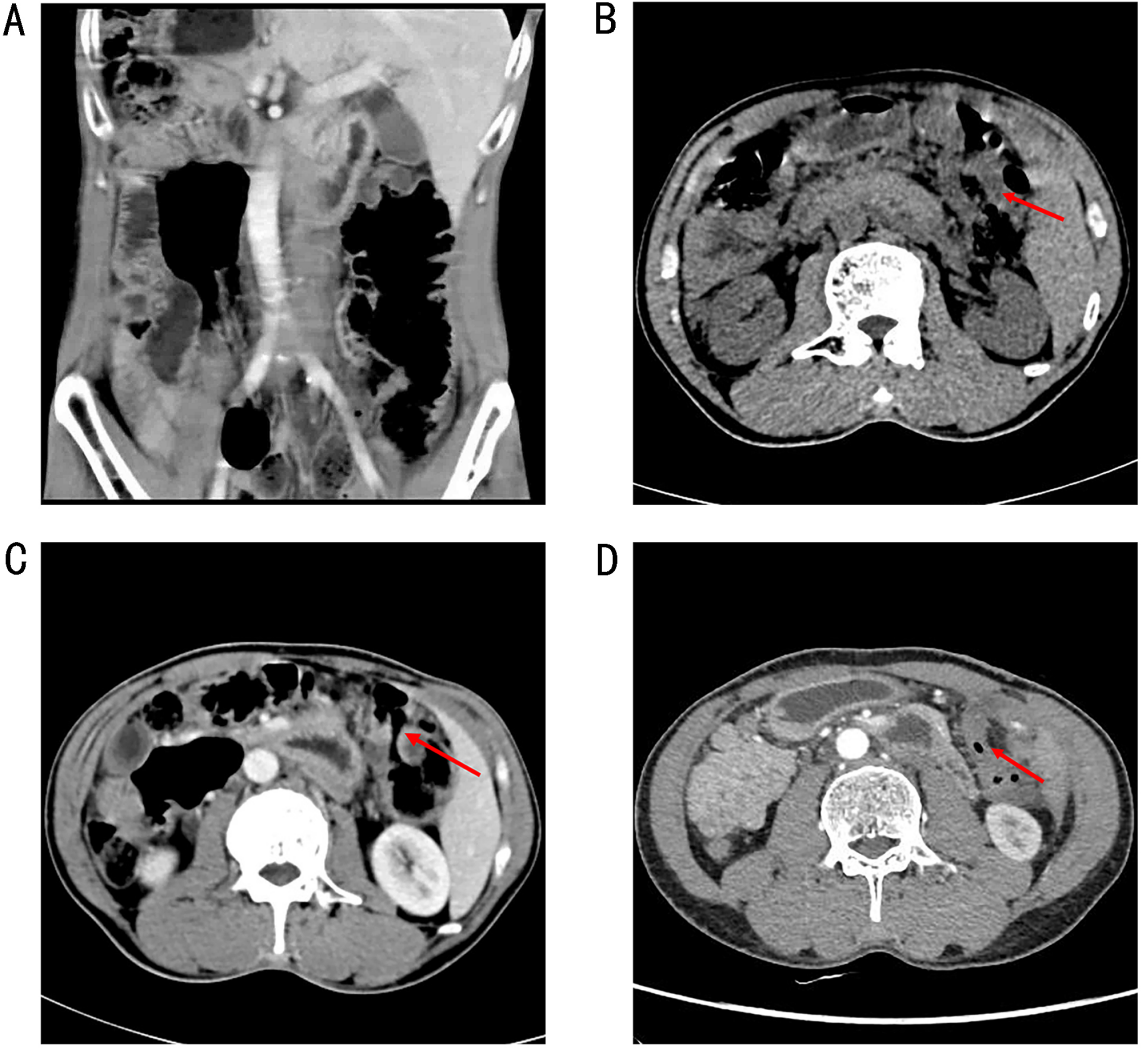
A chest and abdominal CT scan revealed SIT (A) and intestinal wall thickening in the hepatic flexure of the colon (B). CT image showed that the thickening of the colonic hepatic flexure wall was better than before (C) (on April 28, 2021). CT image showed that no abnormal enhancement of the colonic hepatic flexure wall (D) (In September 2021).
